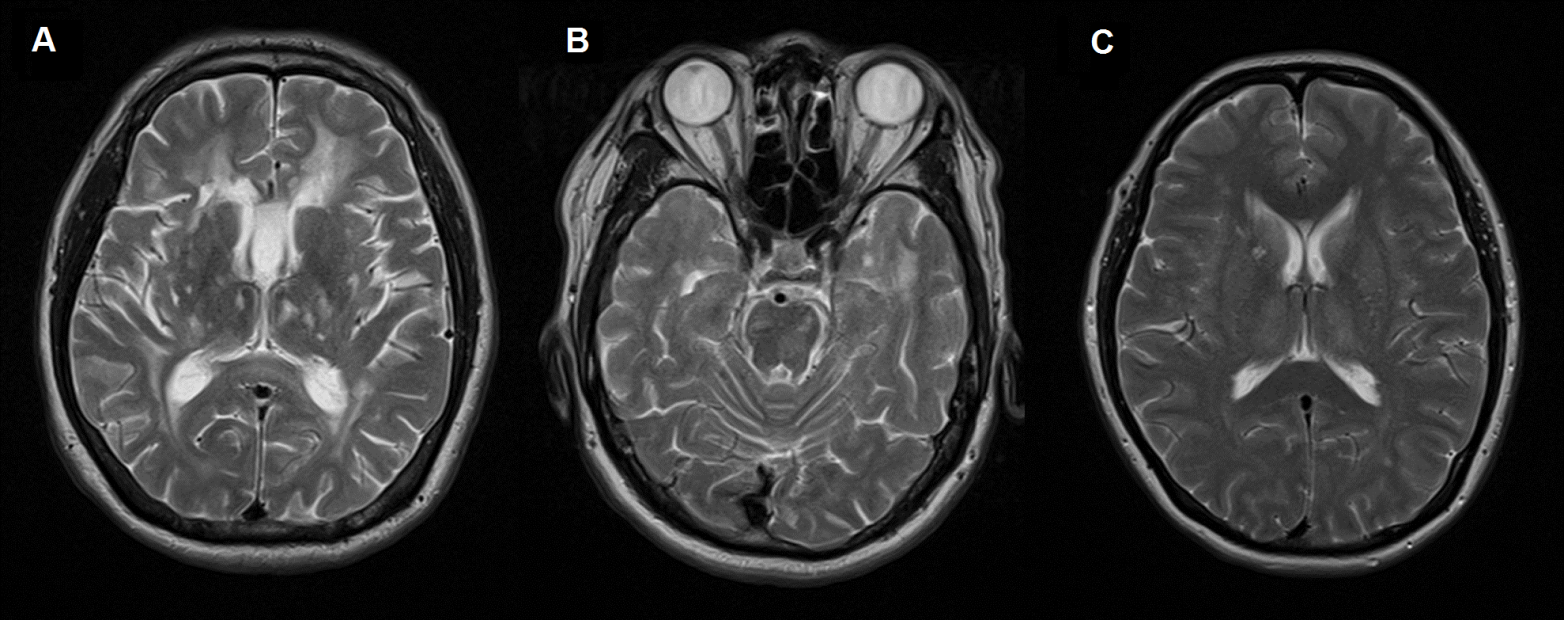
(A): Axial T2W MRI brain of a symptomatic subject (II-9) demonstrates cerebral atrophy. There are confluent hyperintense lesions in the periventricular, subcortical, deep white matter and thalamus, with (B) infra-tentorial and anterior temporal lobe involvement. 3(C): Axial T2W MRI brain of an asymptomatic subject (II-6) demonstrates more discreet deep white matter and periventricular lesions.
